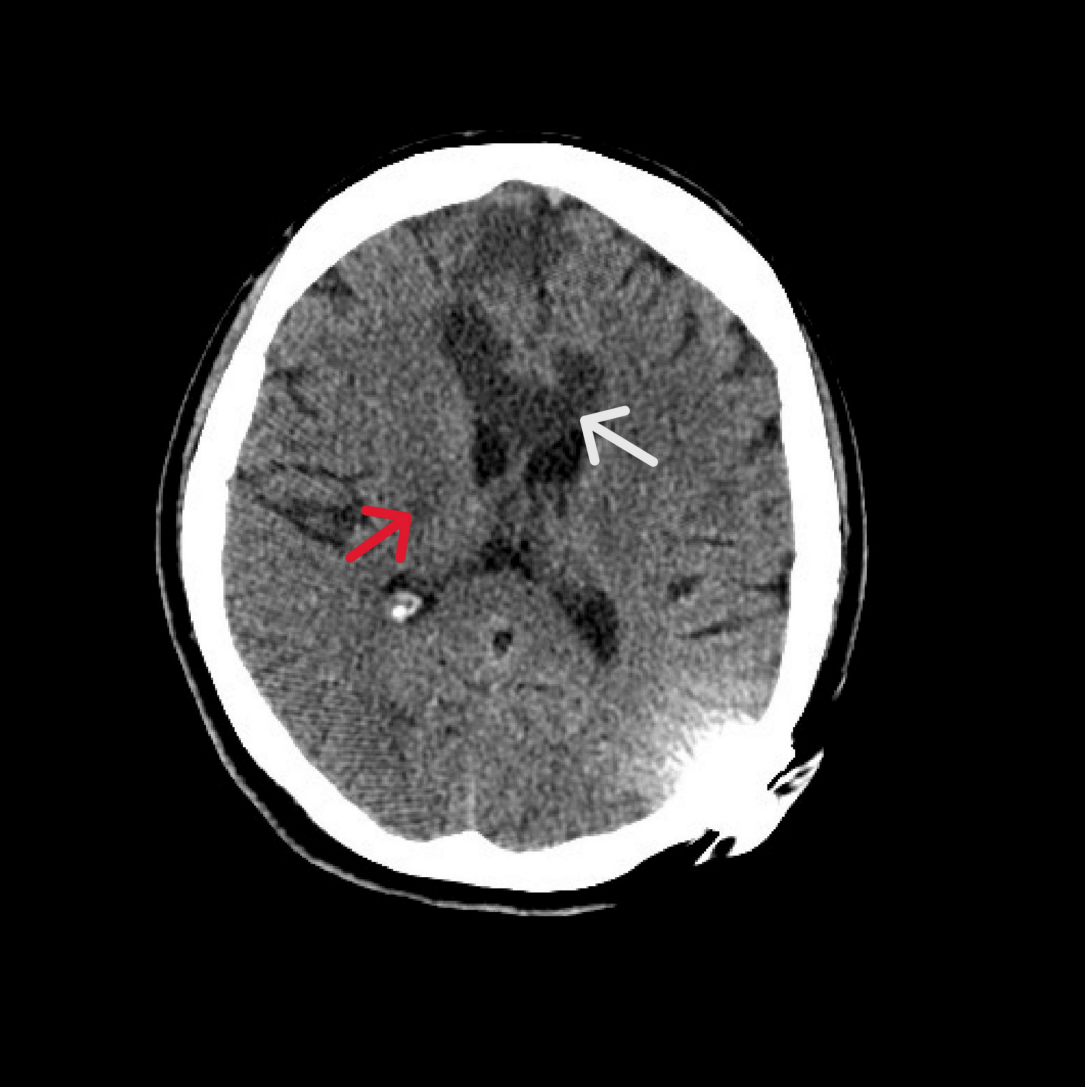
Repeat CT The image shows progressing infarcts within the corpus callosum (white arrow) and bilateral watershed territories (red arrow) CT: computed tomography
